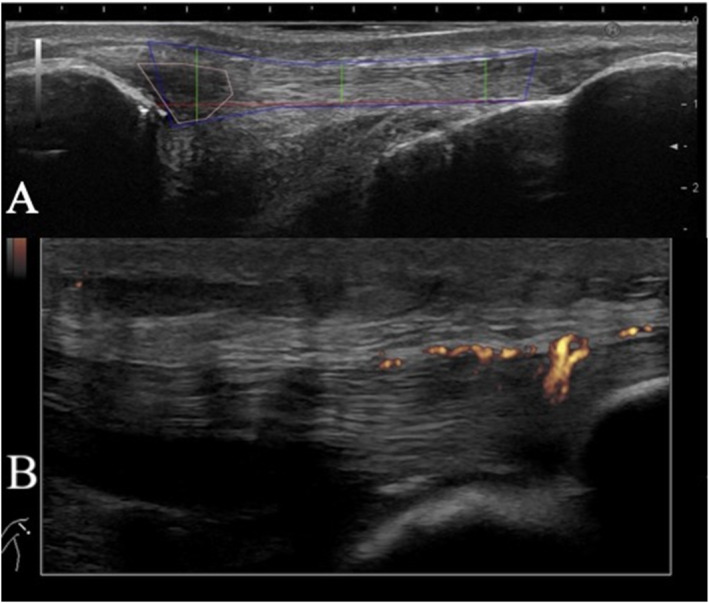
Ultrasonographic evaluation. Sagittal ultrasonographic images of a patellar tendon with left on the image being proximal and right being distal. The upper image (A) visualises the measurements of the thickness and hypoechoic region of the patellar tendon. Firstly, the distance from apex patella to the insertion of the tendon onto the tibial tuberosity was measured (red line); secondly, the anterior–posterior thickness was measured 0.5 cm distal from apex patella at the midpoint and 0.5 cm proximal from the tibial insertion (green lines). Finally, echointensity was measured in the whole tendon (blue outline) and in the hypoechoic region (pink outline). The lower image (B) is an example of the Doppler flow in the patellar tendon and in the peritendinous tissue.
